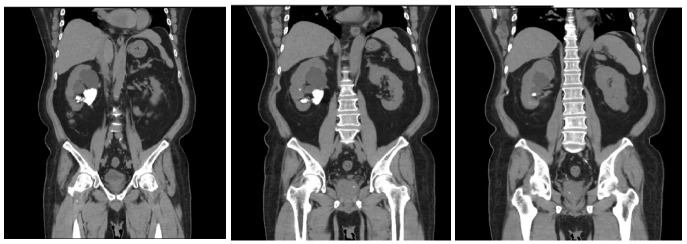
Initial computed tomography (CT) urography. The first CT Urography of the patient shows right staghorn stone with grade 3 hydronephrosis and left kidney cyst.
